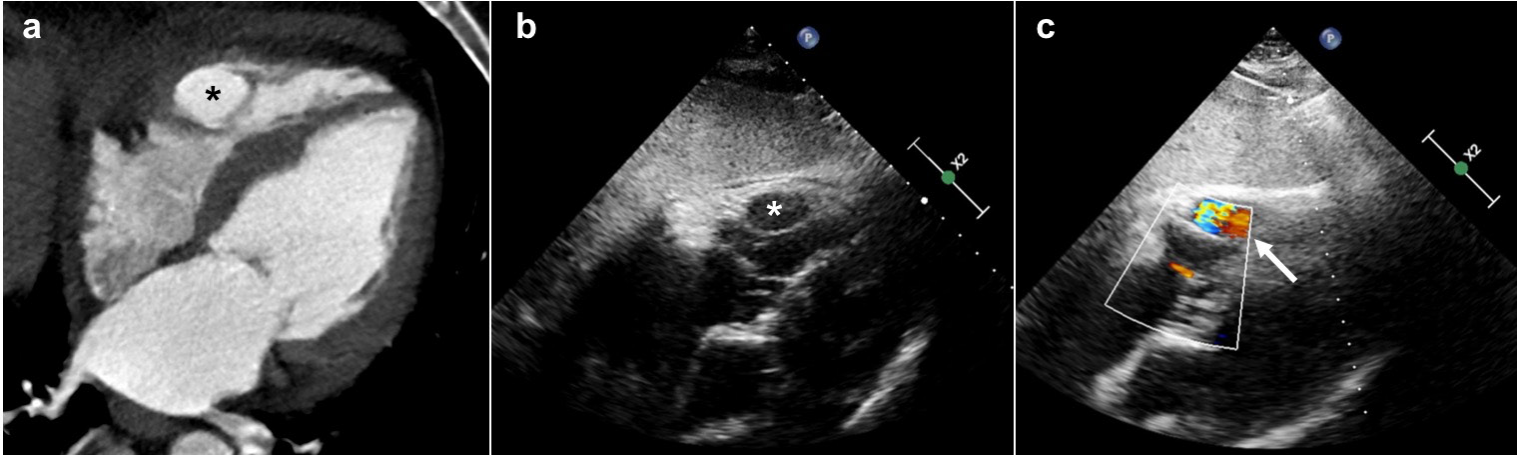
Right ventricle pulsatile chamber (a–b) CCTA and TTE four-chamber view showing the RV intracavitary pulsatile chamber (asterisks). (c) Color-doppler four-chamber view showing the blood flow of the oval cavity contained inside the RV (white arrow). CCTA, cardiac CT angiography; RV, right ventricle; TTE, transthoracic echocardiography.
